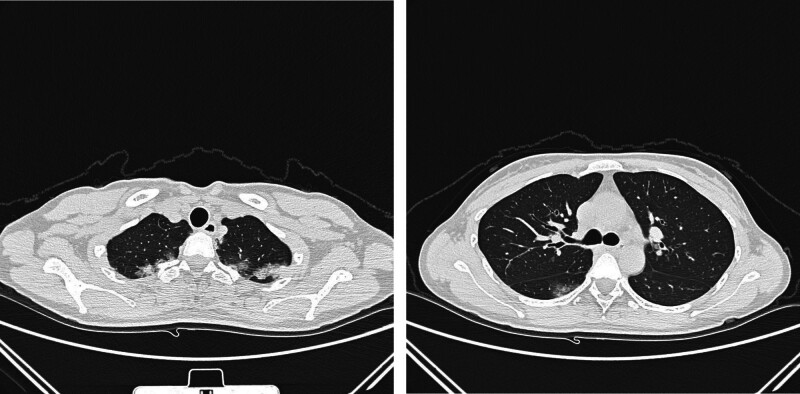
Chest CT showed bilateral patchy shadows in the lung, and the lesions were unchanged as compared to 6 mo prior.
